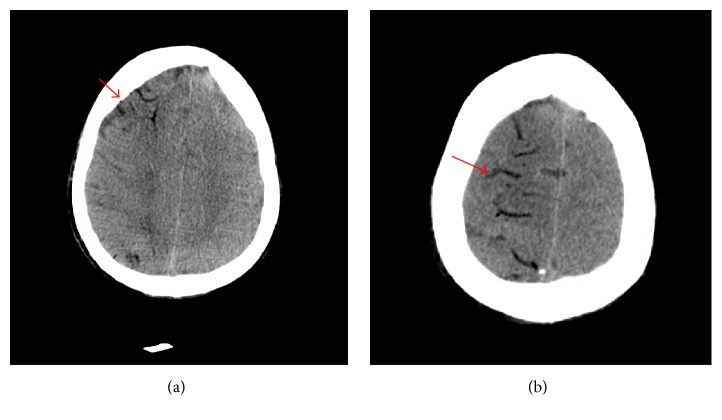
Computed tomography (CT) imaging of the brain showing air accumulation in right hemisphere with edema (arrows).
